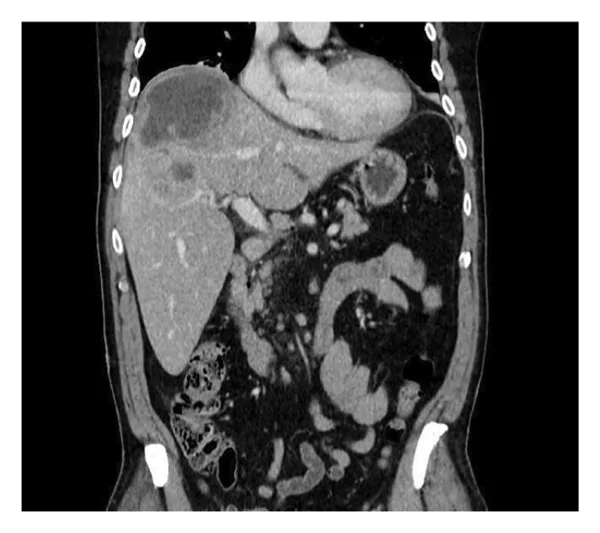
Multiloculated necrotic hepatic lesions demonstrated in the late portal phase on contrast‐enhanced abdominal CT.
